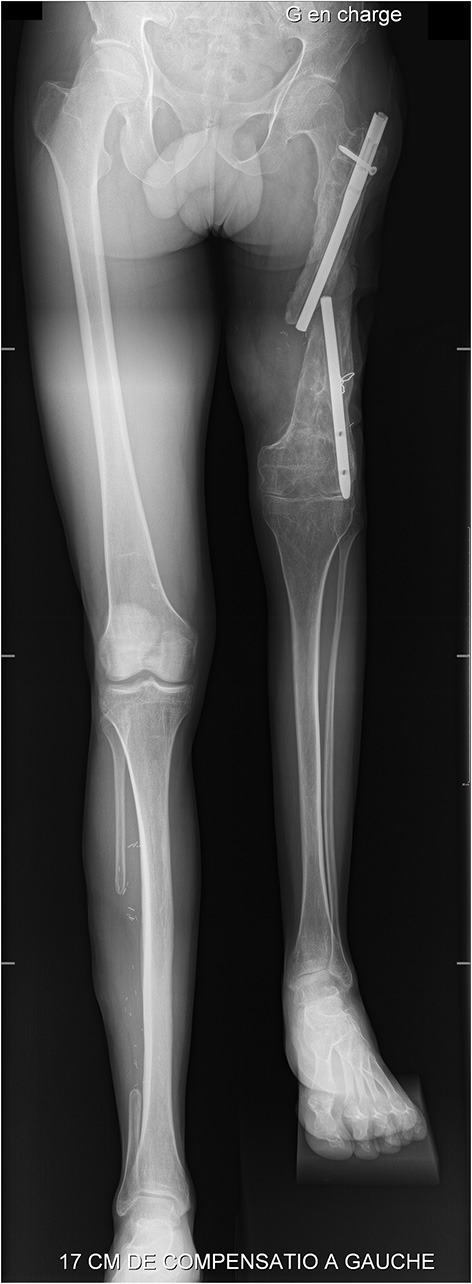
Pre-operative weight-bearing full-length lower limb X-ray showing major limb length discrepancy (17 cm).
